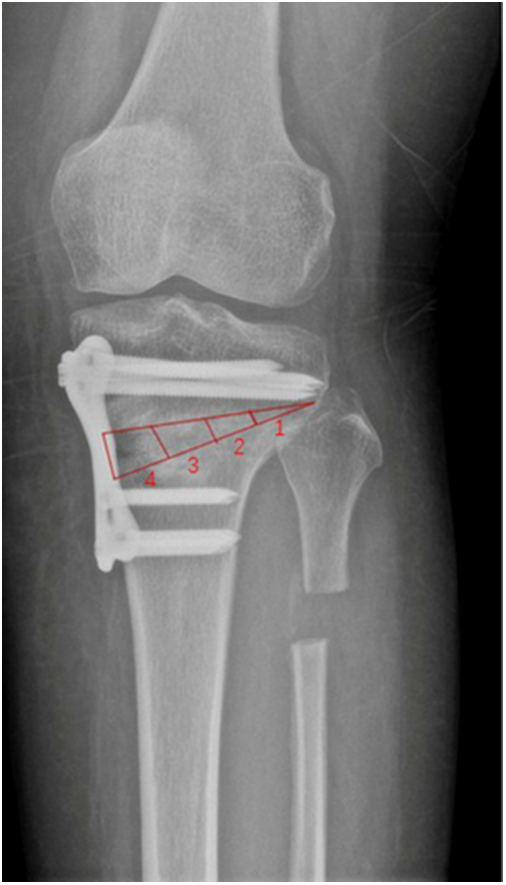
The osteotomy length was divided into four zones on the anteroposterior radiograph. When the bridging callus was more than half of the osteotomy gap (zone 2), it was called radiological bone union.
